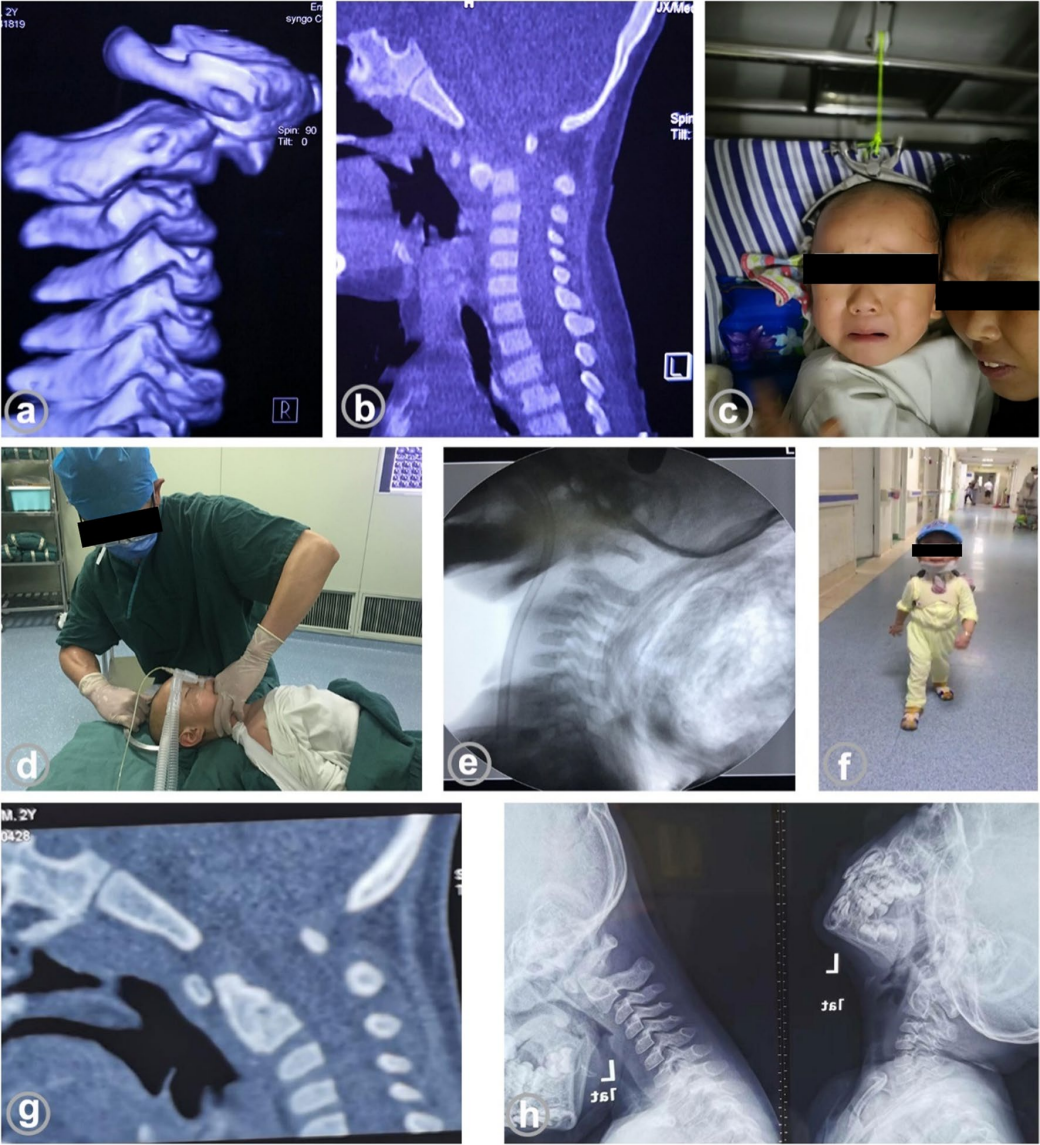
Pre-reduction computed tomography (CT) showing separation of the dentate epiphysis and atlantoaxial dislocation (a, b); the patient was unable to tolerate cranial traction (c); transoral closed reduction was performed under anesthesia (d); fluoroscopy confirmed successful manipulation (e); external fixation of head, neck and chest brace was performed and the patient walked normally (f); 6 months postoperative CT showed closure of the dentate epiphysis (g); 3 years postoperative cervical flexion and extension x-ray showing closure of the dentate epiphysis and no instability of the atlantoaxial spine (h)
